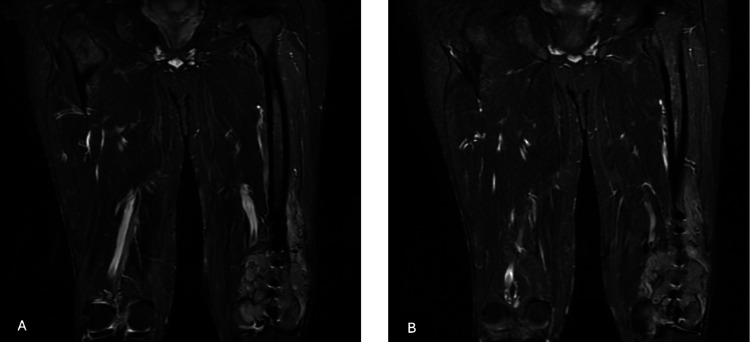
Magnetic resonance images of the patient. (A-B) Coronal views of the patient showing no skip metastasis.
